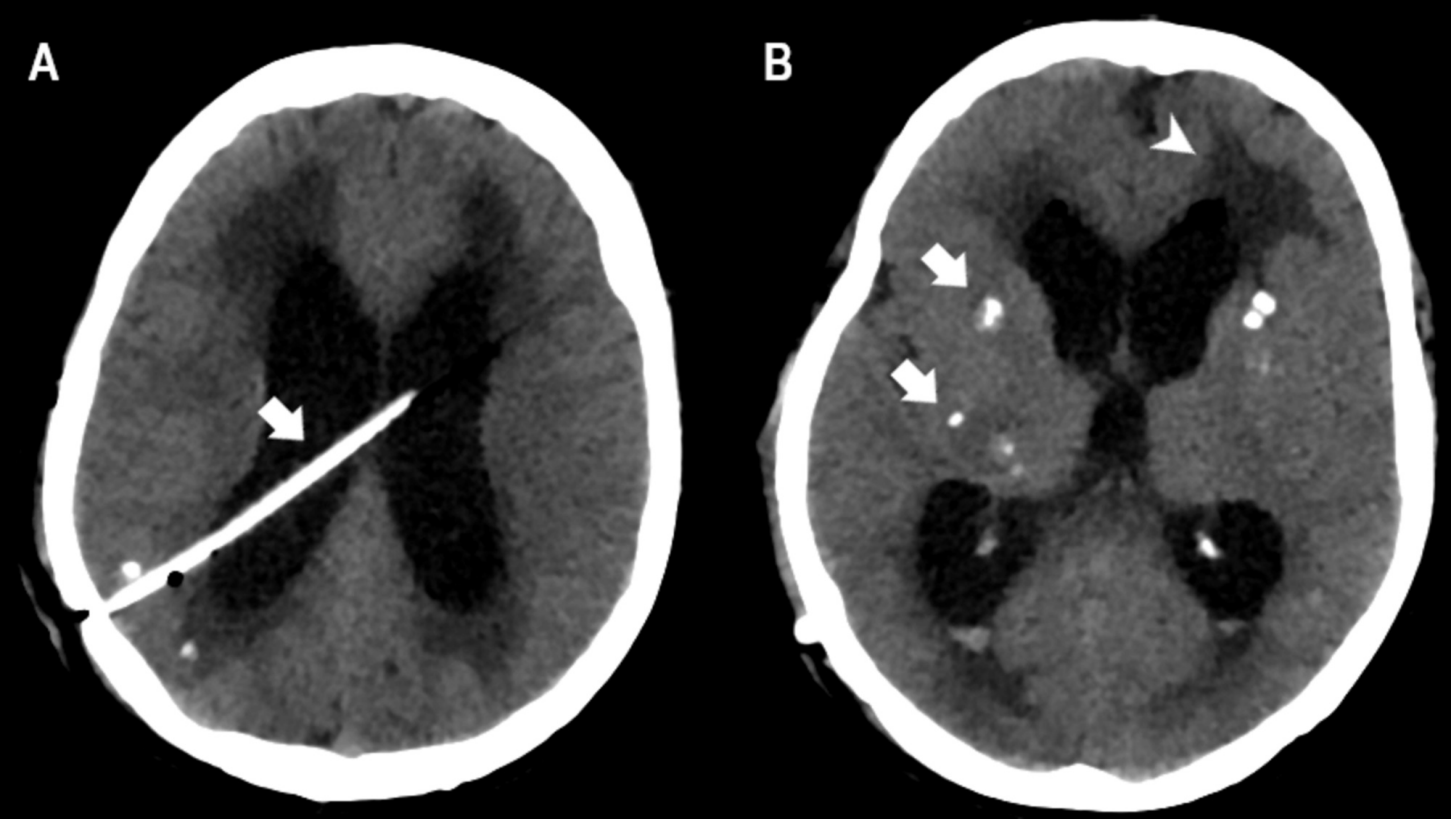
Noncontrast-enhanced cranial CT (A) Noncontrast-enhanced cranial CT showing a well-placed ventricular drain (arrow). (B) Cranial CT showing multiple subcortical calcifications (arrows) associated with transependymal resorption (arrowhead).
